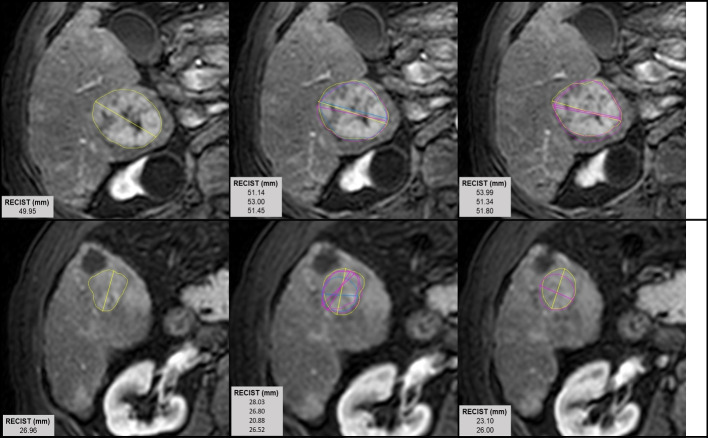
Radiologist-defined RECIST measurements on two example lesions: Lesion codes 19.2 (top row) and 19.3 (bottom row). Radiologists were free to choose the MRI slice on which they indicated the diameter. Within each row, each of the three images depicts a different MRI slice. Diameters drawn by the same radiologist are indicated in the same color (Pink = radiologist A, Yellow = radiologist B, Blue = radiologist C). Lesion contours are included for context. Each image is displayed in the optimum visualization window and phase used for segmentation
